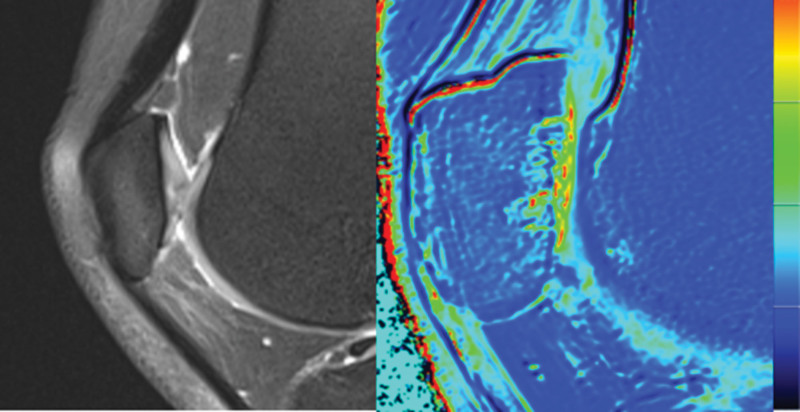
No obvious cartilage injury was detected on conventional MRI images. Functional magnetic resonance imaging showed changes in the biochemical components of the cartilage matrix and cartilage injury. MRI = magnetic resonance imaging.
